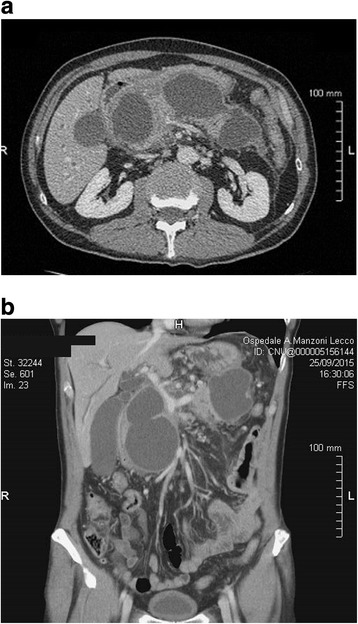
a-b CT scans showed multiple defined hypodense lesions with peripheral contrast enhancing pancreatic areas compatible with cystic lesions. The main one involved the head of the pancreas and measured 12 cm in diameter
